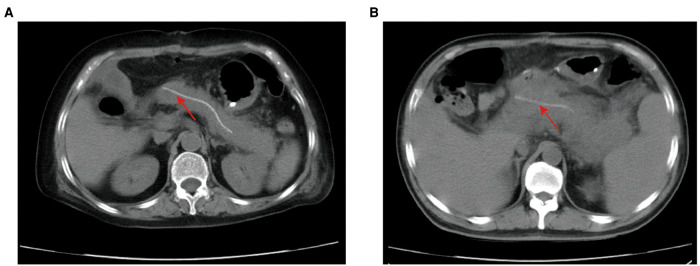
Postoperative CT scans of PPAP. (A) Acute edematous pancreatitis after PD: the boundary of the remnant pancreas is coarse, extensive exudation and inflammatory change around the remnant pancreas, not the surgical field. (B). Acute necrotizing pancreatitis after PD: the borderline of remnant pancreas is not distinct, inflammatory changes and exudate surrounding the remnant pancreas, necrosis change in the pancreatic parenchyma and the peripancreatic tissue. Red arrow: internal pancreatic duct stent. PPAP, postoperative acute pancreatitis; PD, pancreaticoduodenectomy.
